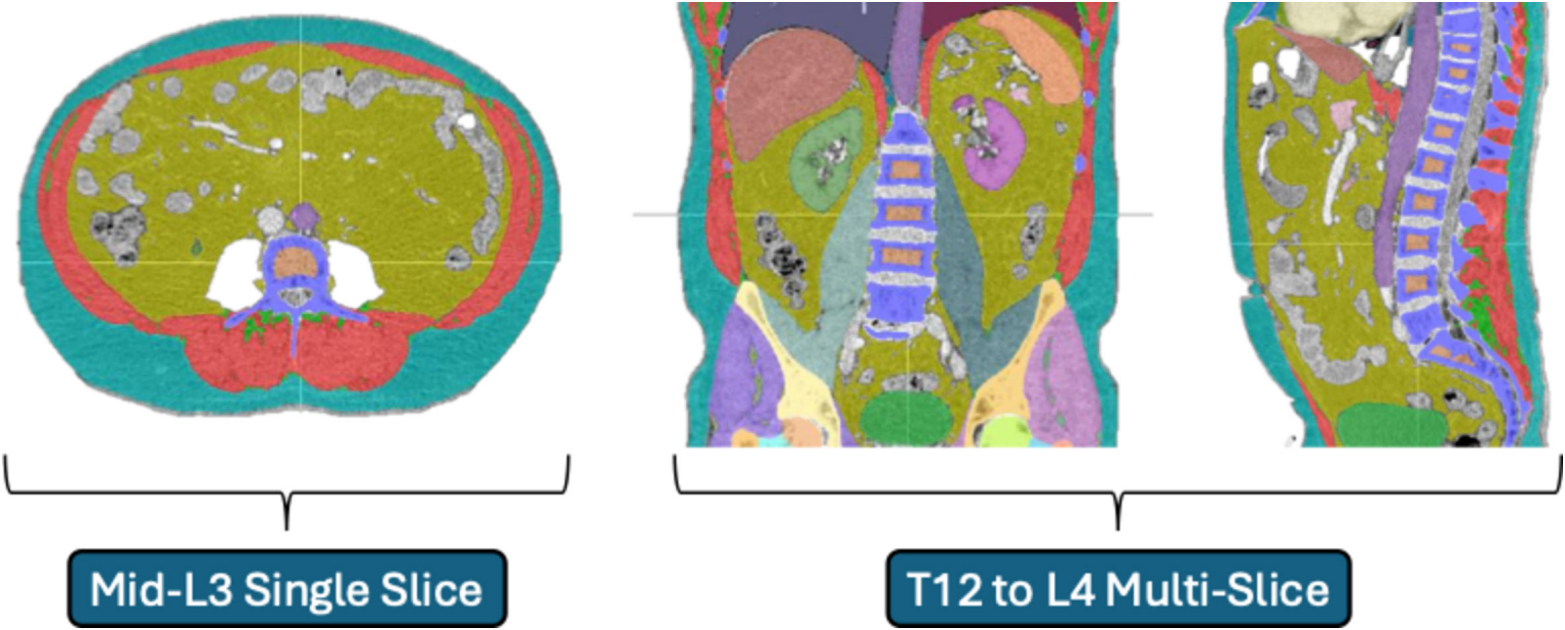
Comparison of body composition analysis at mid‐L3 and T12–L4 vertebral Levels. Note the segmented CT imaging displayed on coronal and sagittal planes (right) extends beyond T12–L4 region of interest analysed.
